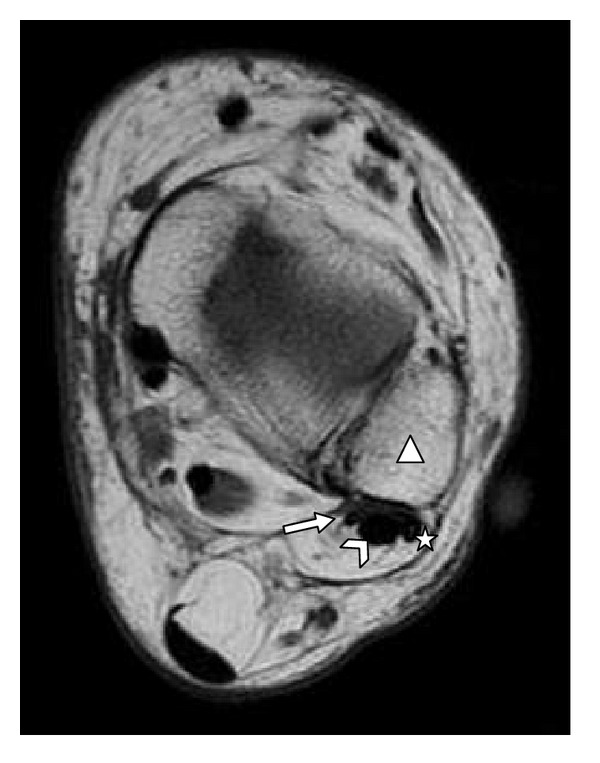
MRI, transverse T1 18/660 of left ankle. Triangle: left fibula; star: fibularis longus tendon; arrowhead: fibularis brevis tendon; arrow: fibularis quartus tendon.
